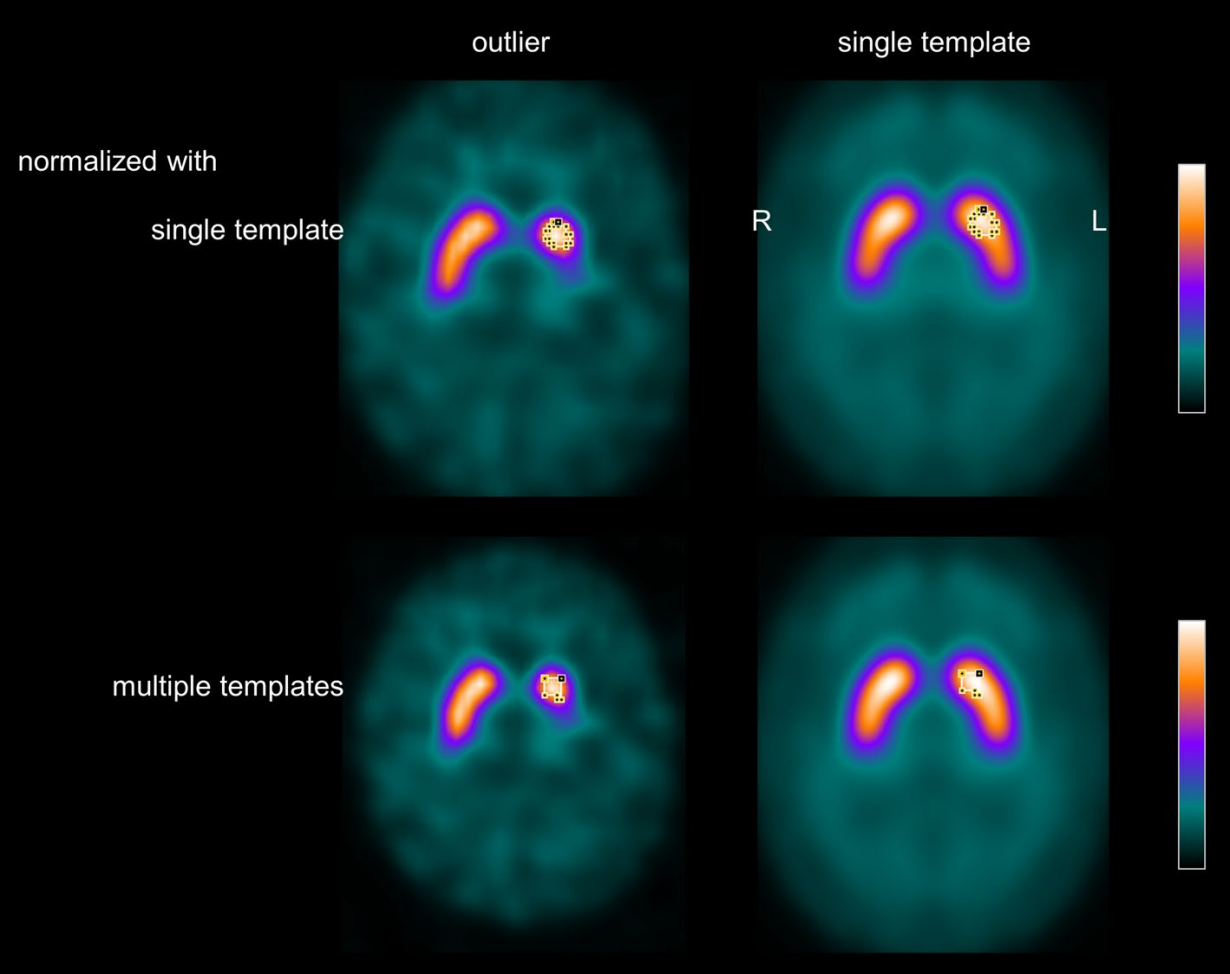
Spatial shifting of the striatum by stereotactical normalization with the single template. The figure shows a transversal image of the DAT-SPECT of the outlier (red dot in Fig. 6) after stereotactical normalization with the single normal template together with this template (top row). The 80%-isodensity contour of the outlier’s left striatum was copied to the normal template. The bottom row shows the same for the DAT-SPECT image of the outlier after stereotactical normalization with multiple templates
